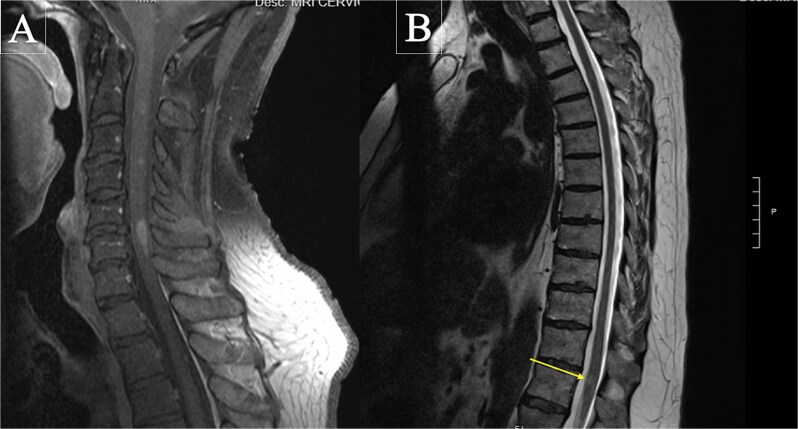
Sagittal T2-weighted magnetic resonance imaging of the spine. (A) Cervical spine demonstrating multiple intramedullary T2-hyperintense lesions. (B) Thoracic spine showing short-segment intramedullary T2-hyperintense lesions (arrow).
